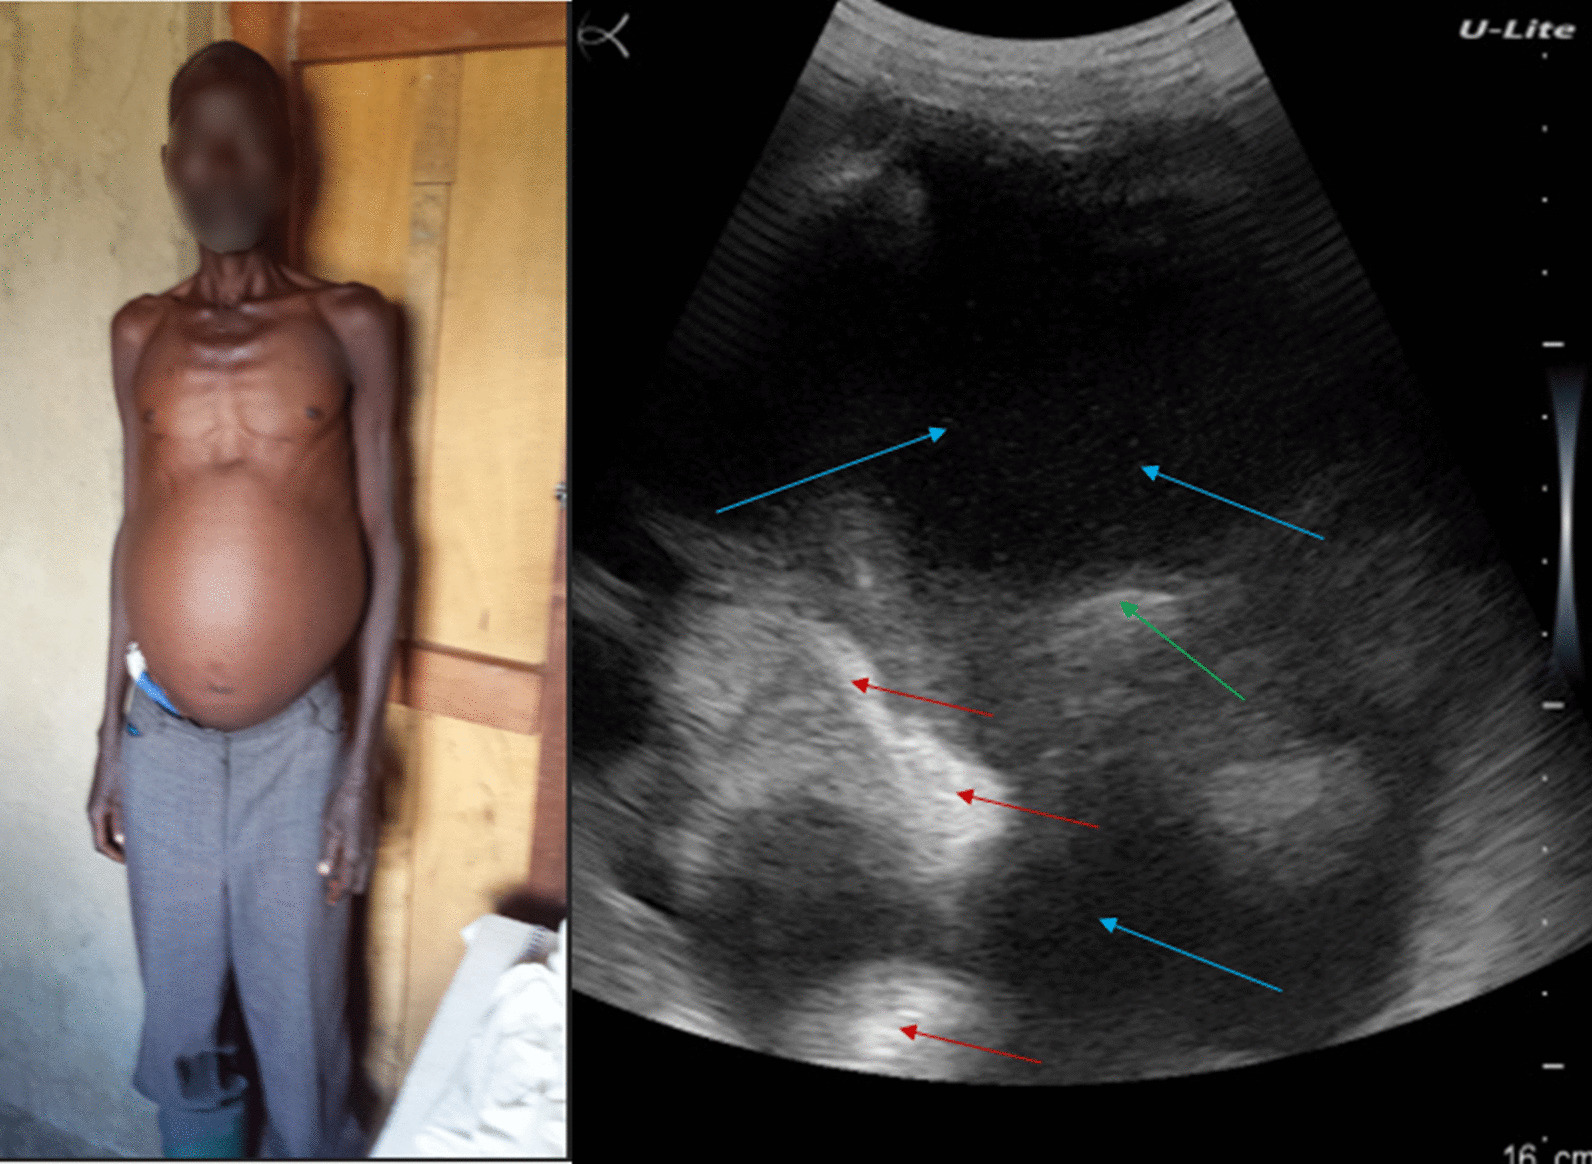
Patient #3 (left). Ultrasound examination (right) showing shrunken cirrhotic liver with distorted architecture, nodular surface, and heterogeneous echotexture of the parenchyma (red arrow), regenerating nodules (green arrow), and ascites (blue arrow)
